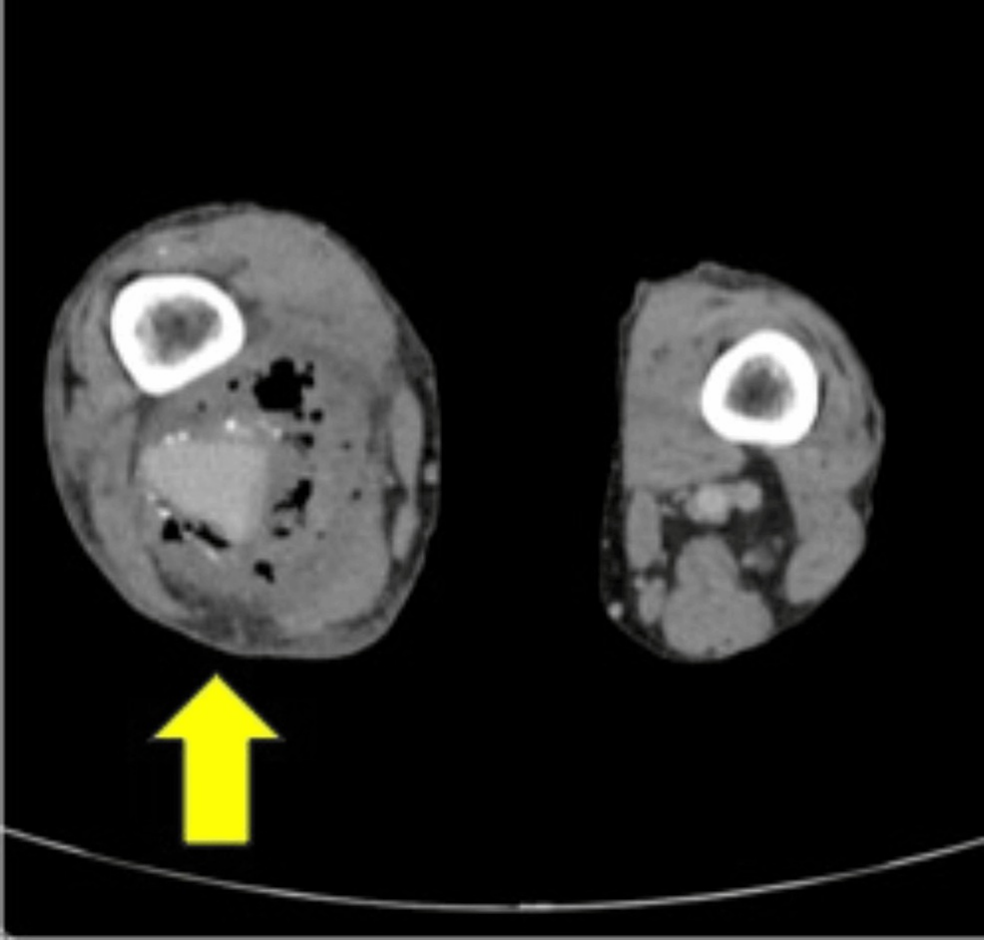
Preoperative horizontal computed tomography (CT) scan of the area around the PAA It shows a right popliteal aneurysm with free air in the surrounding tissue (arrow). PAA: popliteal artery aneurysm
